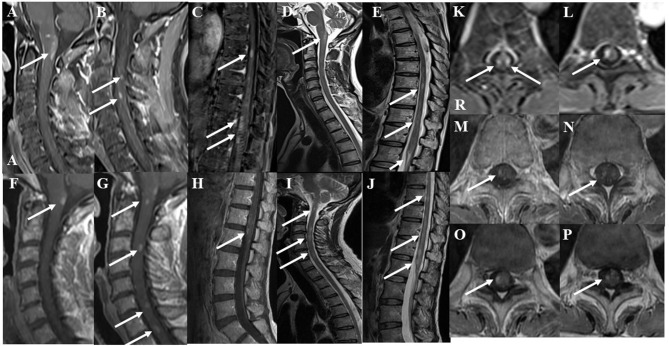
Gadolinium-enhanced spinal magnetic resonance imaging (MRI) findings at the initial attack. The lower “A” represents “anterior” in (A). The “R” represents “right” in (K). (A–C) Post-contrast fat-suppression T1-weighted images showed multiple abnormal foci in the medulla and spinal cord at the initial attack. (D,E) T2-weighted images showed multiple abnormal foci in the medulla and spinal cord at the initial attack. (F–H) Post-contrast T1-weighted MRI of the cervicothoracic and lumbar spine after two courses of high-dose steroid pulse therapy. Abnormal enhancement was slightly decreased, but new enhanced lesions appeared in the spinal cord at the 3rd, 6th, and 7th levels of the cervical spine. (I,J) T2-weighted MRI of the cervicothoracic and lumbar spine after two courses of high-dose steroid pulse therapy. T2-weighted images showed patchy lesions with a marked increase in signals in the spinal cord at the 3rd and 6th levels of the cervical spine and at the 9, 10, and 12th levels of the thoracic spine. (K,L) Axial fat-suppression T1-weighted images showed enhanced lesions at the 9 and 12th levels of the thoracic spine at the initial attack. (M,N) After two courses of high-dose steroid pulse therapy, follow-up T1-weighted MRI showed that abnormal enhancement was decreased. (O,P) After three courses of high-dose steroid pulse therapy and 5 plasmapheresis treatments, follow-up MRI showed that the enhanced foci were diminished but still present.
